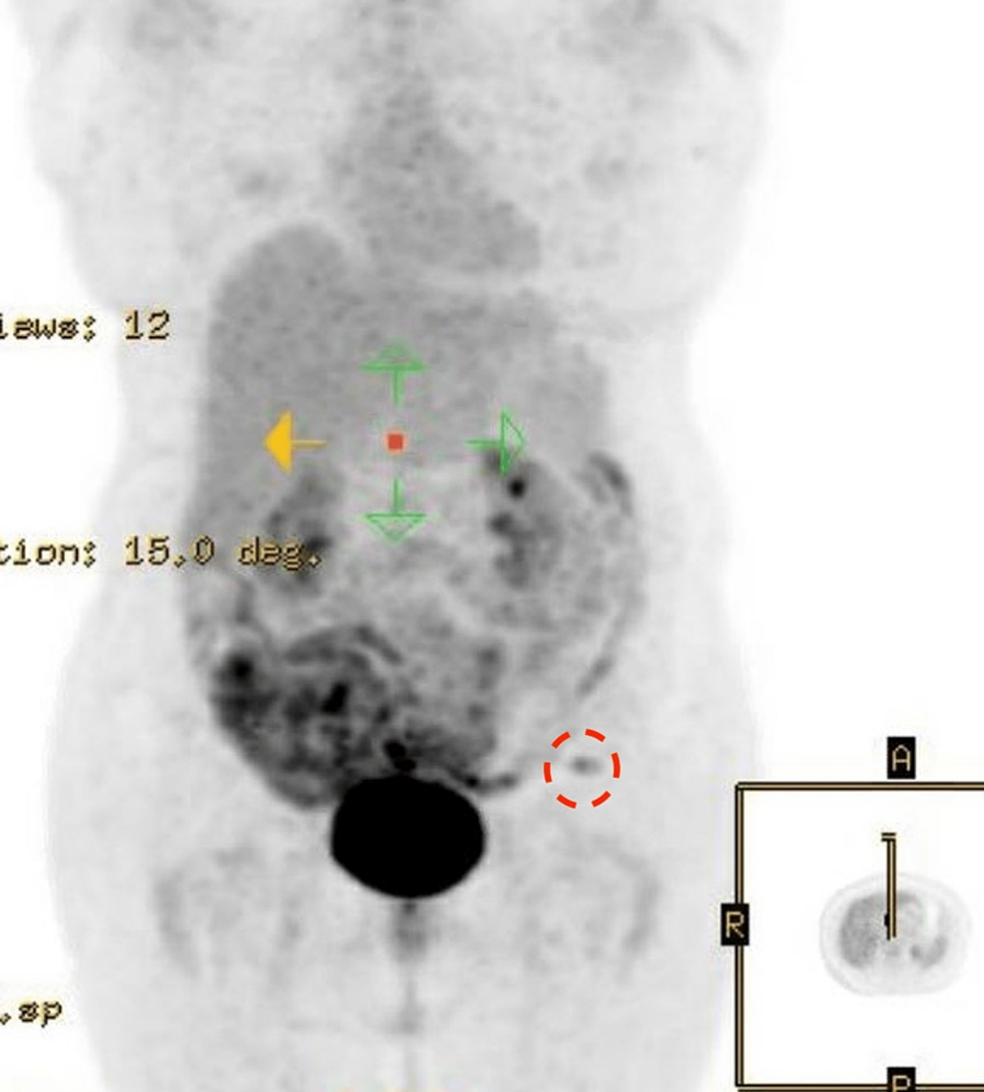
PET scan showing 2 cm left groin mass with mild FDG-avid activity
